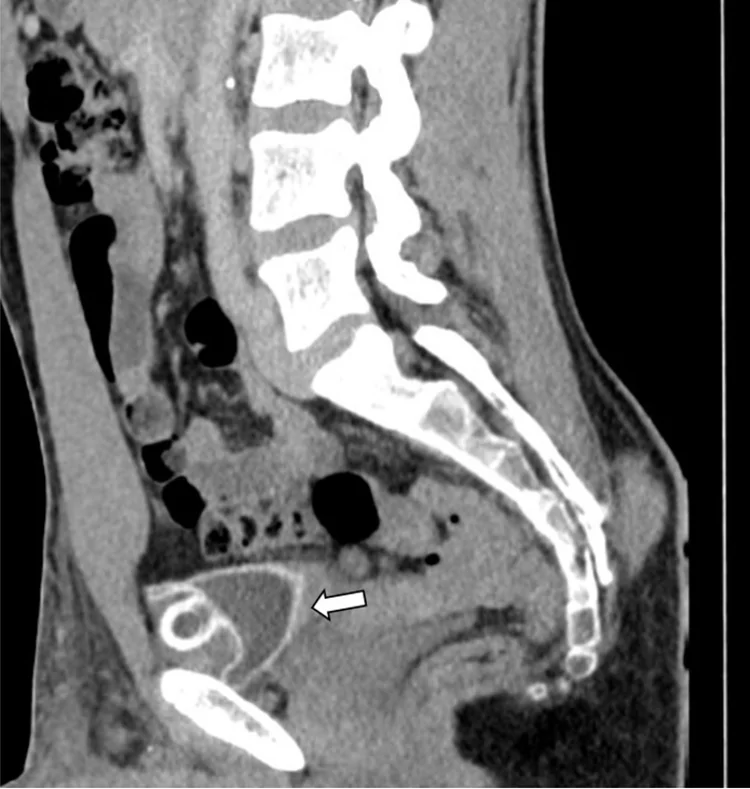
Sagittal reformatted CT image shows the reservoir of a three-piece penile prosthesis in the space of Retzius (arrow)
